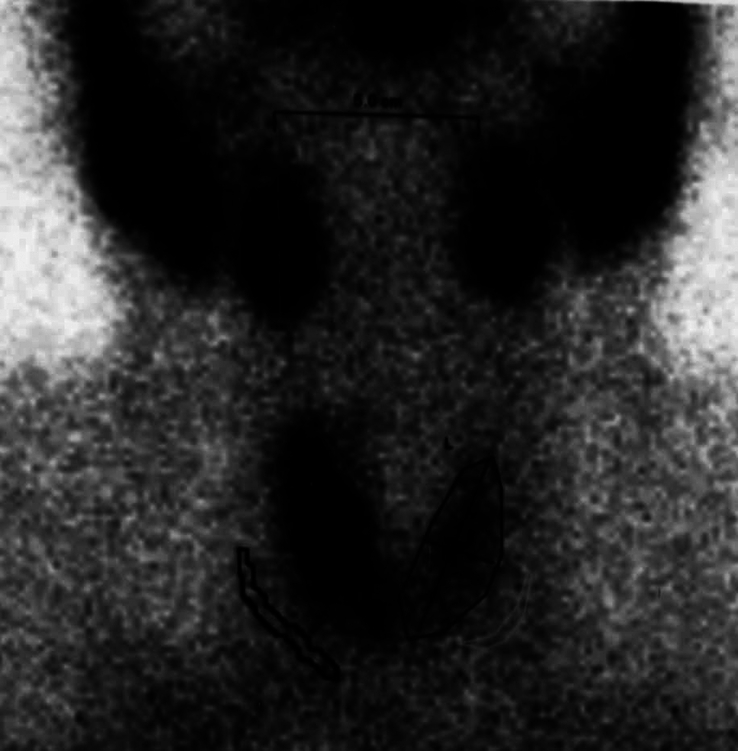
Ultrasonography and 99mTC pertechnetate thyroid scintigraphy.
